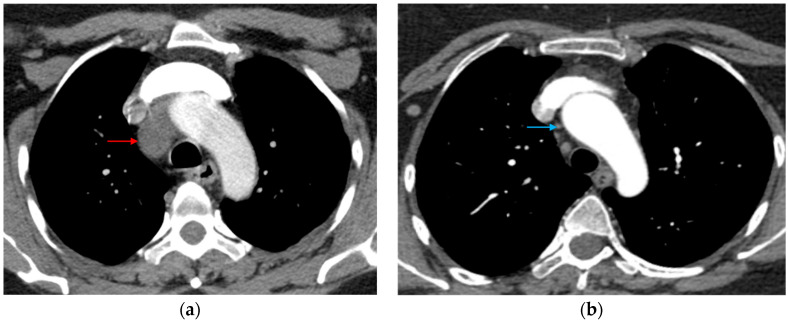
High-riding aorta superior pericardial recess. (a) Axial CTA image demonstrates a lobulated fluid attenuation structure in the right upper paratracheal region (red arrow) corresponding to a high-riding superior pericardial recess. (b) A comparative axial CTA image at the same level in a patient with no pericardial recess abnormalities (blue arrow).
